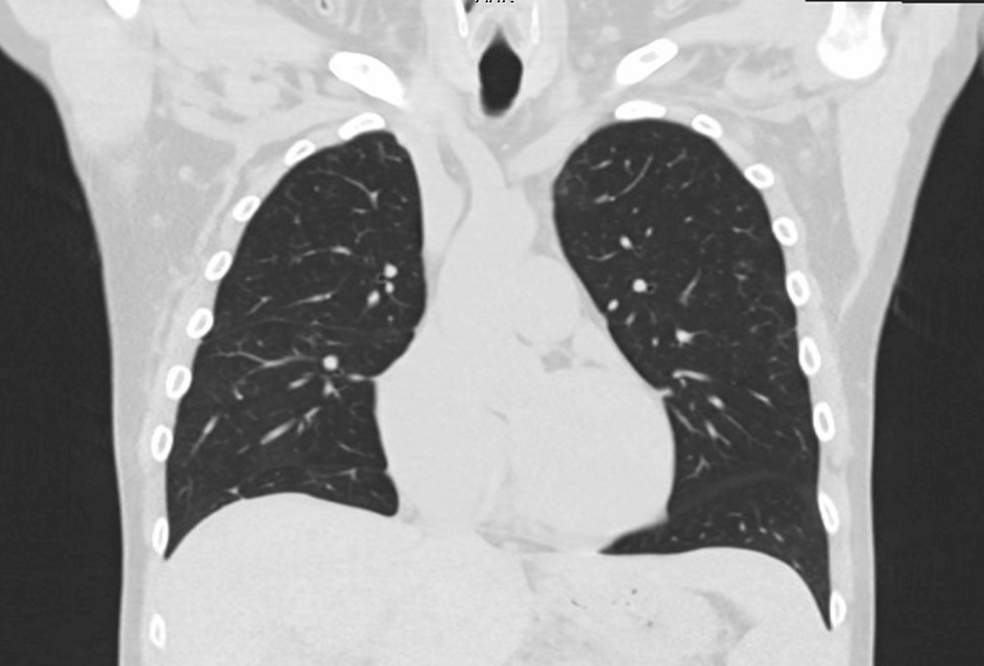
Chest CT scan (coronal view) reassessment six weeks after hospital discharge.
